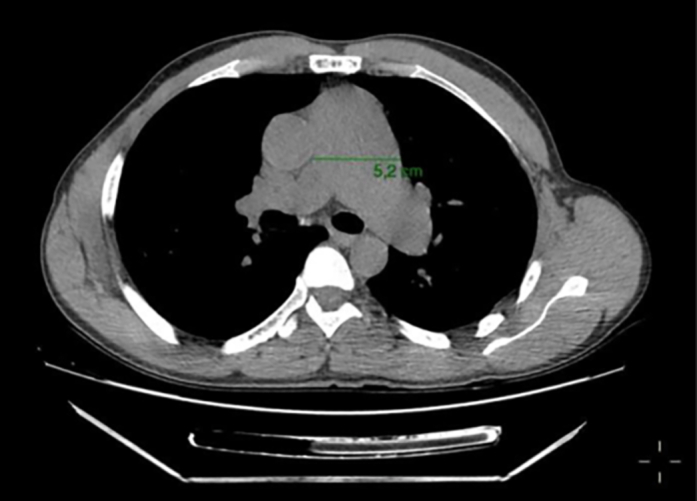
Chest Computed Tomography Scan Showing Main Pulmonary Artery Aneurysm With a 52-mm Diameter
